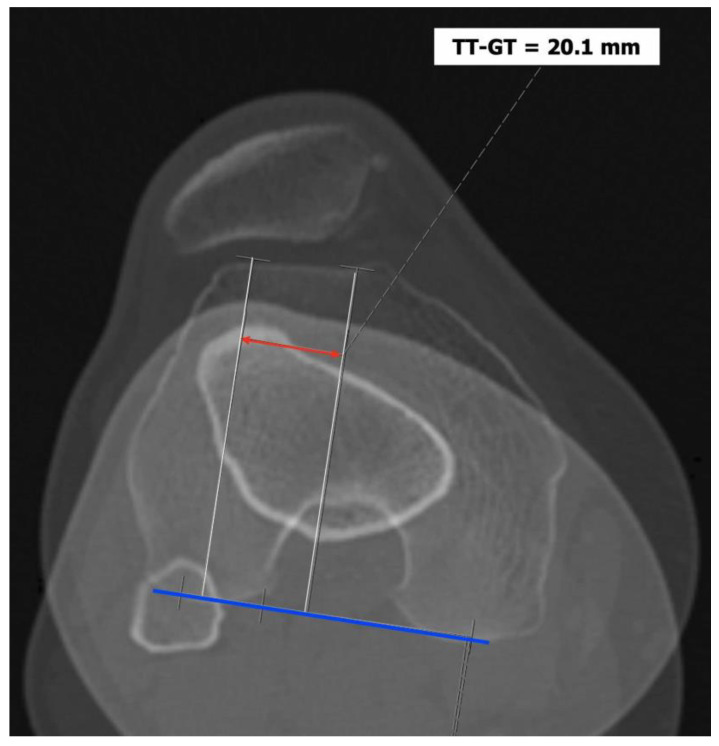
CT scan with pathological TT-TG of 20.1 mm.
